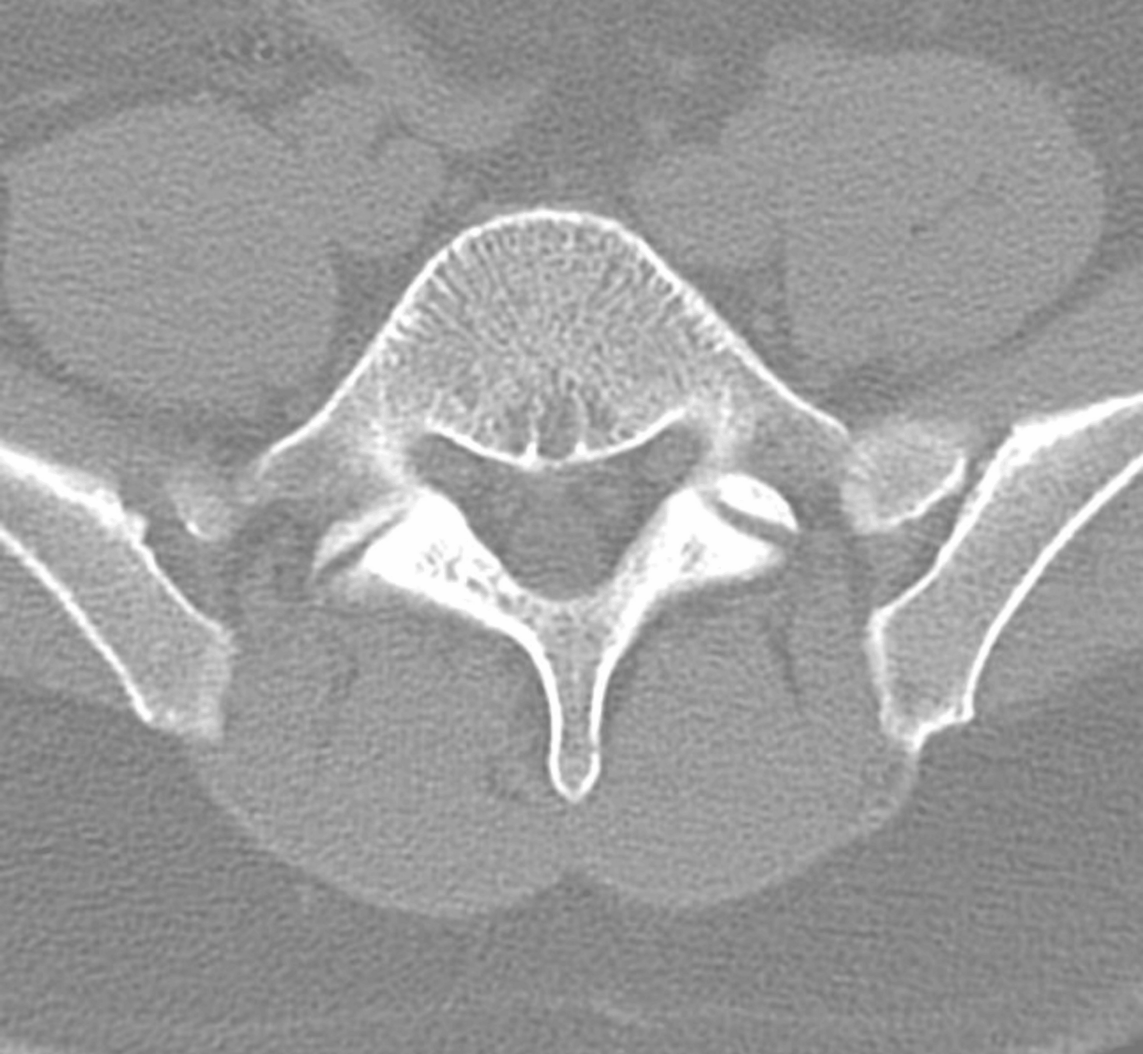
Axial computed tomography image at the L5–S1 level demonstrating degenerative facet arthropathy without evidence of acute fracture, significant spinal canal stenosis, or acute osseous abnormality.
